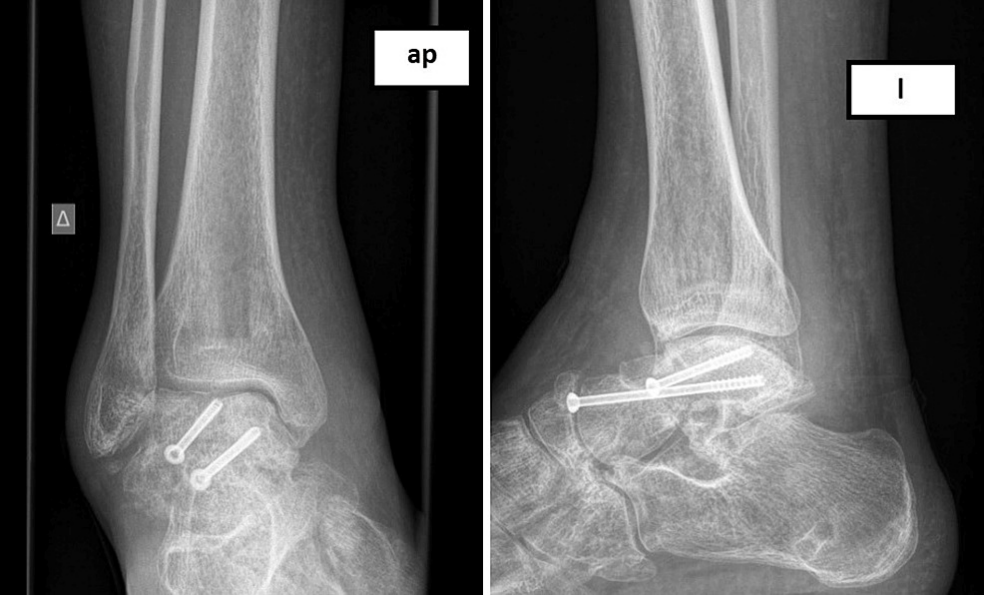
Three months after the initial surgery. The external fixation device has been removed. Anteroposterior (AP) and lateral (L) radiograph showing varus malunion
